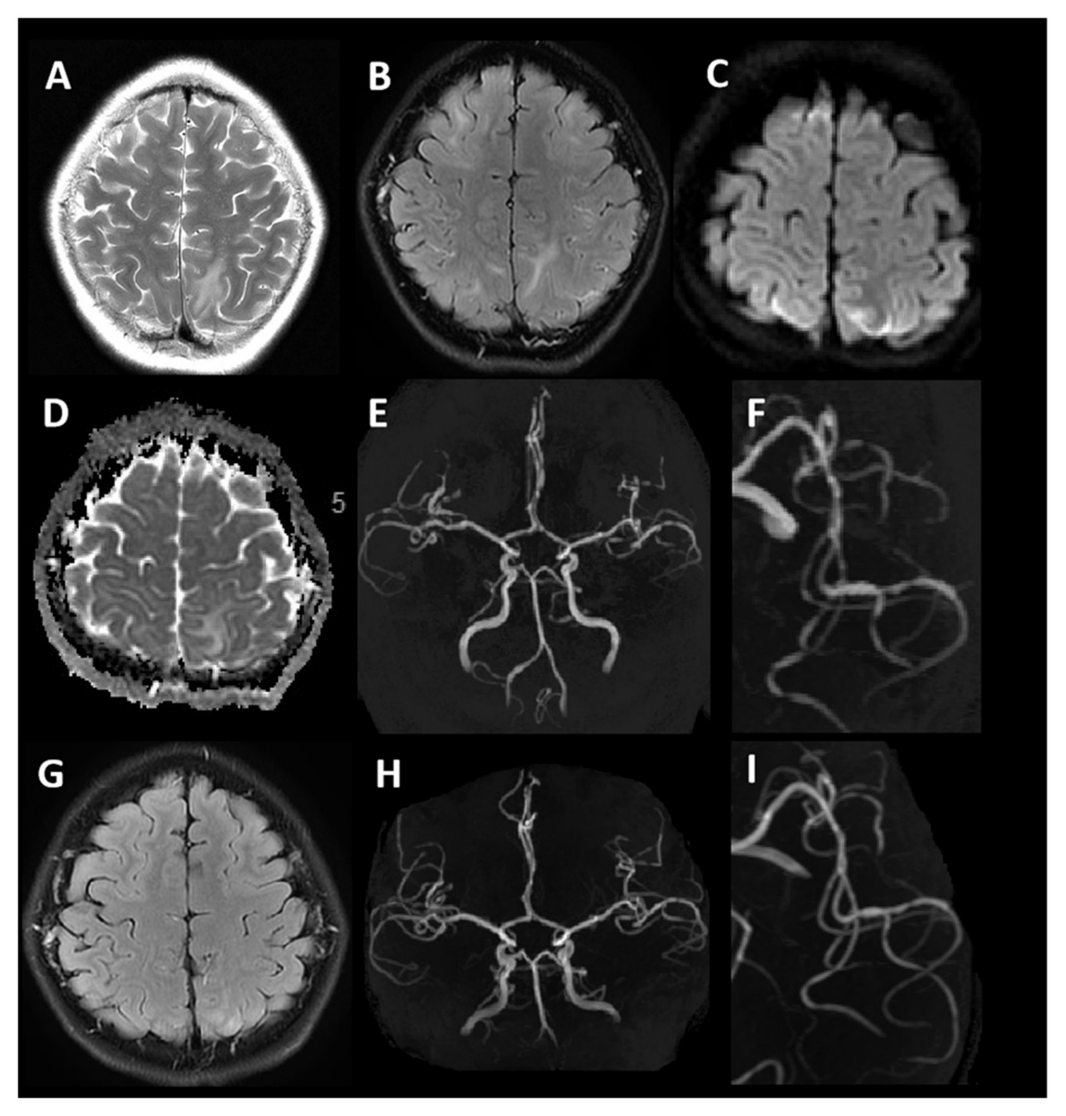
Typical MRI findings in PRES coexisting with RCVS. A 12-year-old female with lupus nephritis. T2WI and T2 -FLAIR imaging (A,B) show hyperintense signals involving bilateral subcortical white matter of the frontal, parietal, and temporal lobes. DWI (C) shows hyperintense signals in bilateral frontoparietal lobes. The corresponding ADC map (D) shows no diffusion restriction. MRA (E,F) demonstrates segmental vasoconstriction in the left middle cerebral artery branch. Follow-up MRI after 10 days (T2-FLAIR) (G) demonstrates complete resolution of signal abnormalities. MRA (H,I) demonstrates normalization of vessel irregularity.
